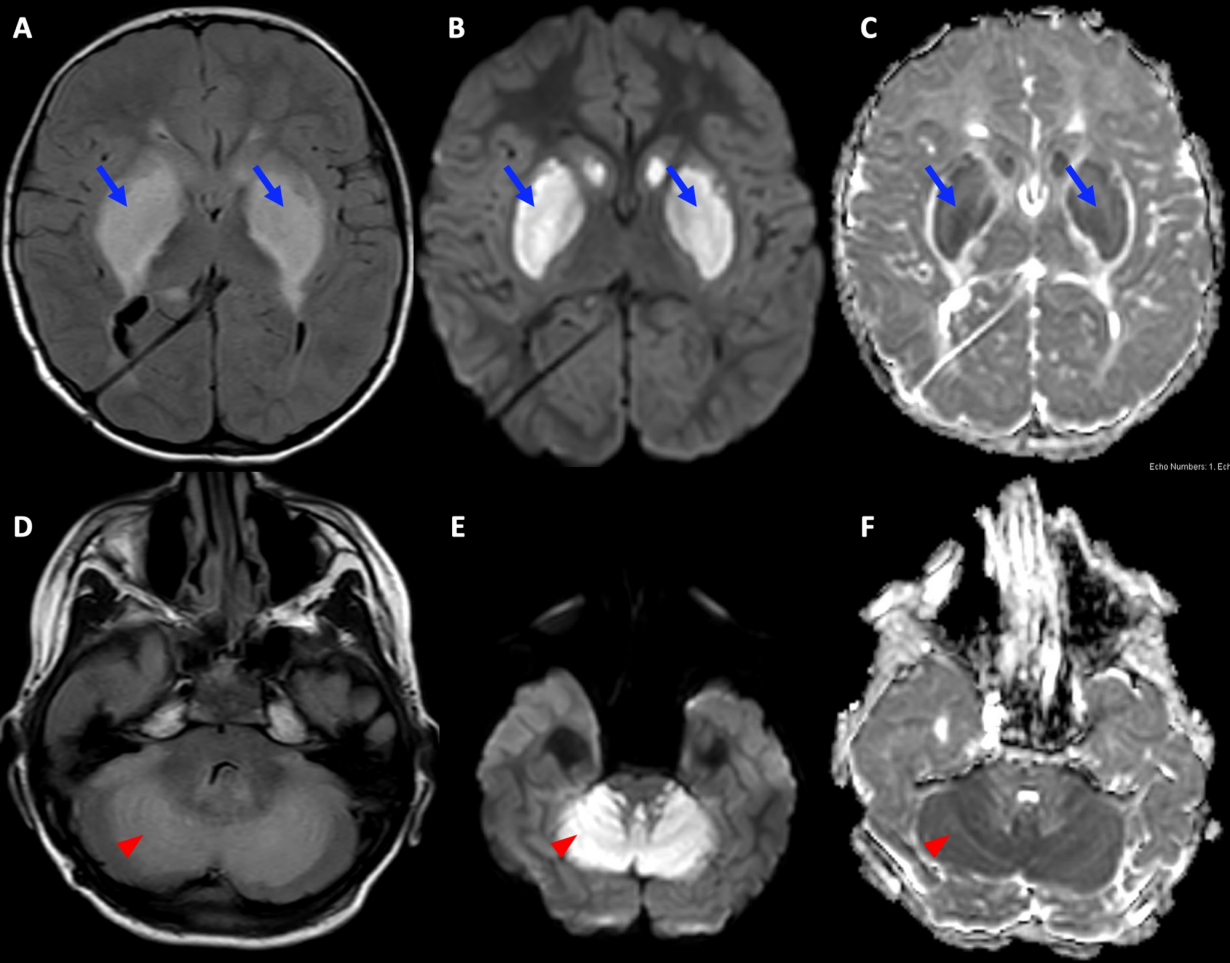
Follow-Up MRI Findings Obtained Three Days After Initial Imaging Images 2A, 2B, 2C: T2 fluid-attenuated inversion recovery (FLAIR), isotropic diffusion-weighted, and apparent diffusion coefficient (ADC) map images demonstrated increased areas of diffusion restriction in the basal ganglia (blue arrows). Images 2D, 2E, 2F: T2 FLAIR, isotropic diffusion-weighted, and ADC map images demonstrated increased areas of diffusion restriction in the cerebellum (red arrowheads).
